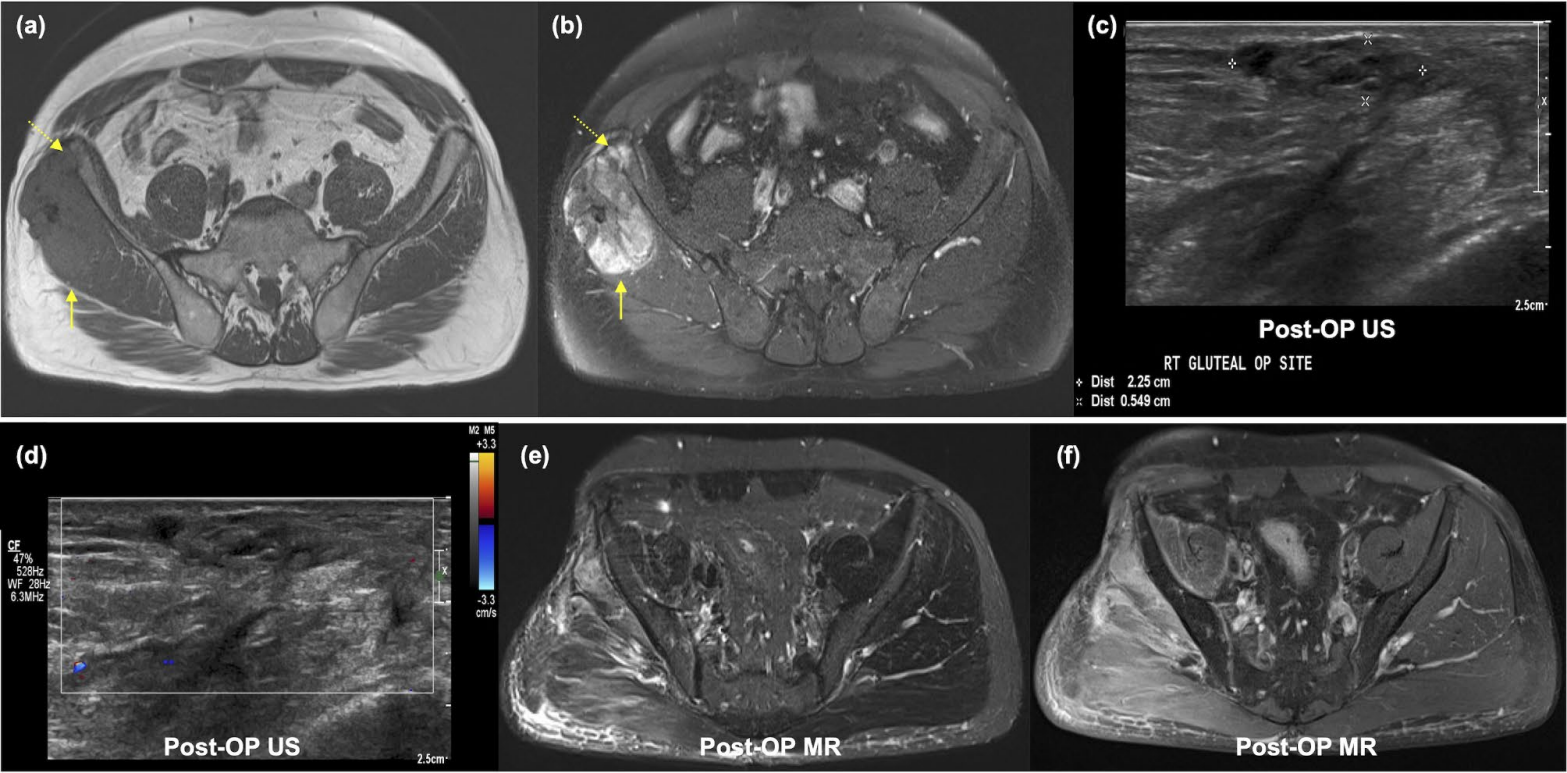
False-positive USG in a 62-year-old male with undifferentiated pleomorphic sarcoma. (a,b) Axial pre- and post-enhanced T1-weighted images revealed a large, heterogeneously enhancing mass (arrows) at the right gluteus medius and minimus with focal extension to the right iliac bone (dashed arrows). (c) On his postoperative USG, taken 9 months after wide resection, a 2.2-cm irregular ovoid-shaped lesion with internal heterogeneous echogenicity was observed in the subcutaneous layer of the right gluteus region. (d) No vascularity was detected based on color Doppler USG. As a result of these USG findings, the MRI examination was rescheduled. (e,f) Axial fat-saturated T2-weighted and enhanced T1-weighted images revealed increased signal intensity and enhancement at the right gluteus medius, gluteus minimus, and iliacus, indicative of radiation-induced myositis. However, no definite nodular-enhancing lesion corresponding to the USG findings was identified
